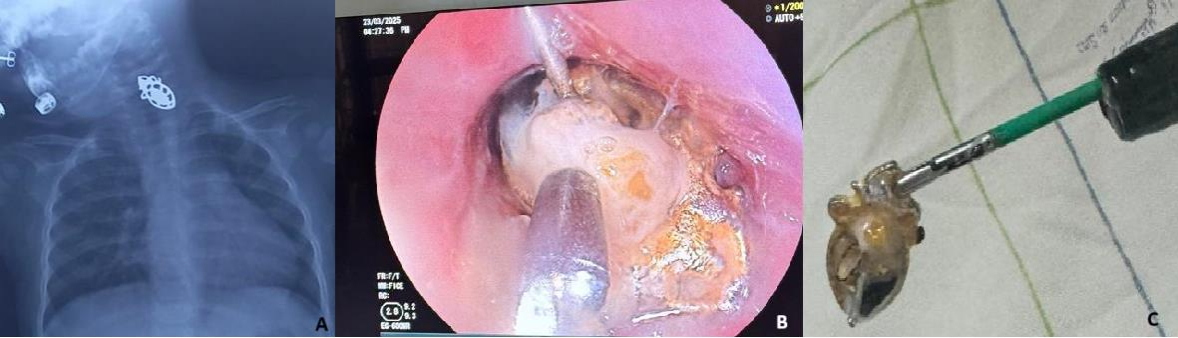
A) chest X-ray showing the foreign body in the upper third of the esophagus; B) endoscopic image showing the extraction of the earring covered in food debris by forceps; C) image of the extracted earring
